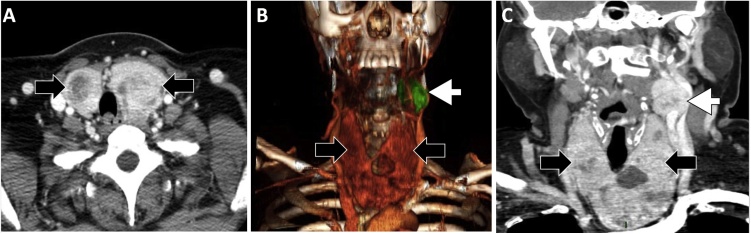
Goiter (black arrows) and paraganglioma (white arrows) in a computerized tomography angiography (CTA). A) Axial view demonstrates displacement of the airway to the right, B) Three-dimensional reconstruction, C) Coronal view.
